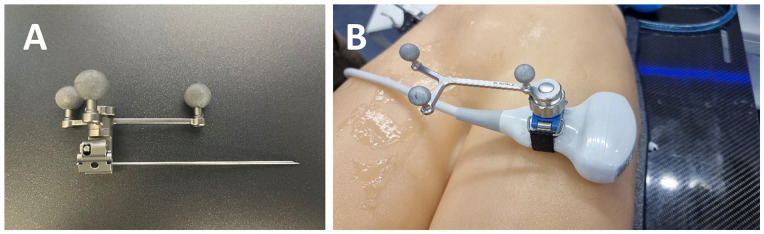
(A) Needle with a reference array. (B) Ultrasound probe with a reference array necessary for sonography-assisted registration.
